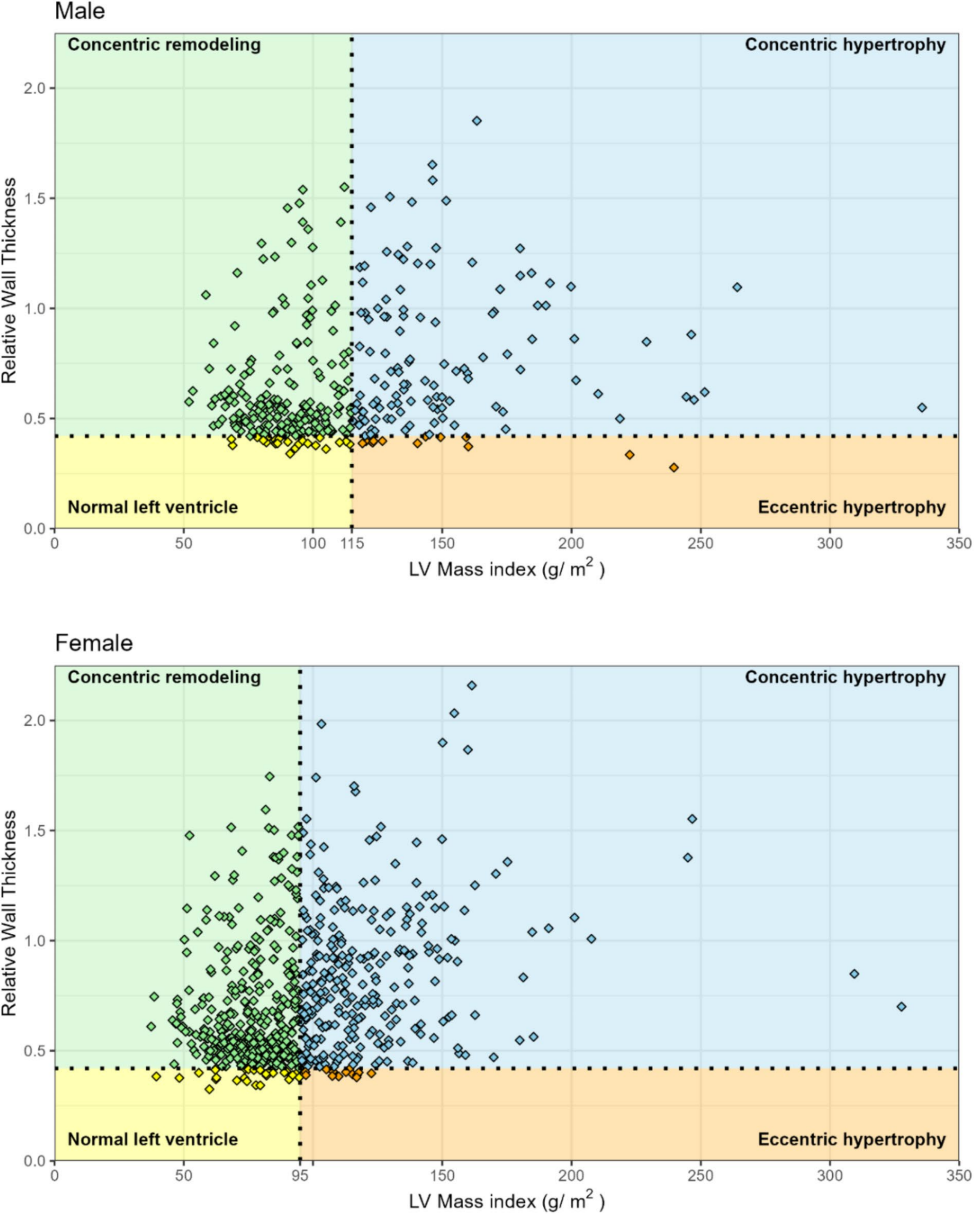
Left ventricular mass index (LVMI) plotted against relative wall thickness (RWT) in all participants with echocardiography. The vertical dotted line represents the cut-off values for normal LVMI (< 95 g/m^2^ in women and < 115 g/m^2^ in men) and the horizontal dashed line for RWT (normal < 0.42).
